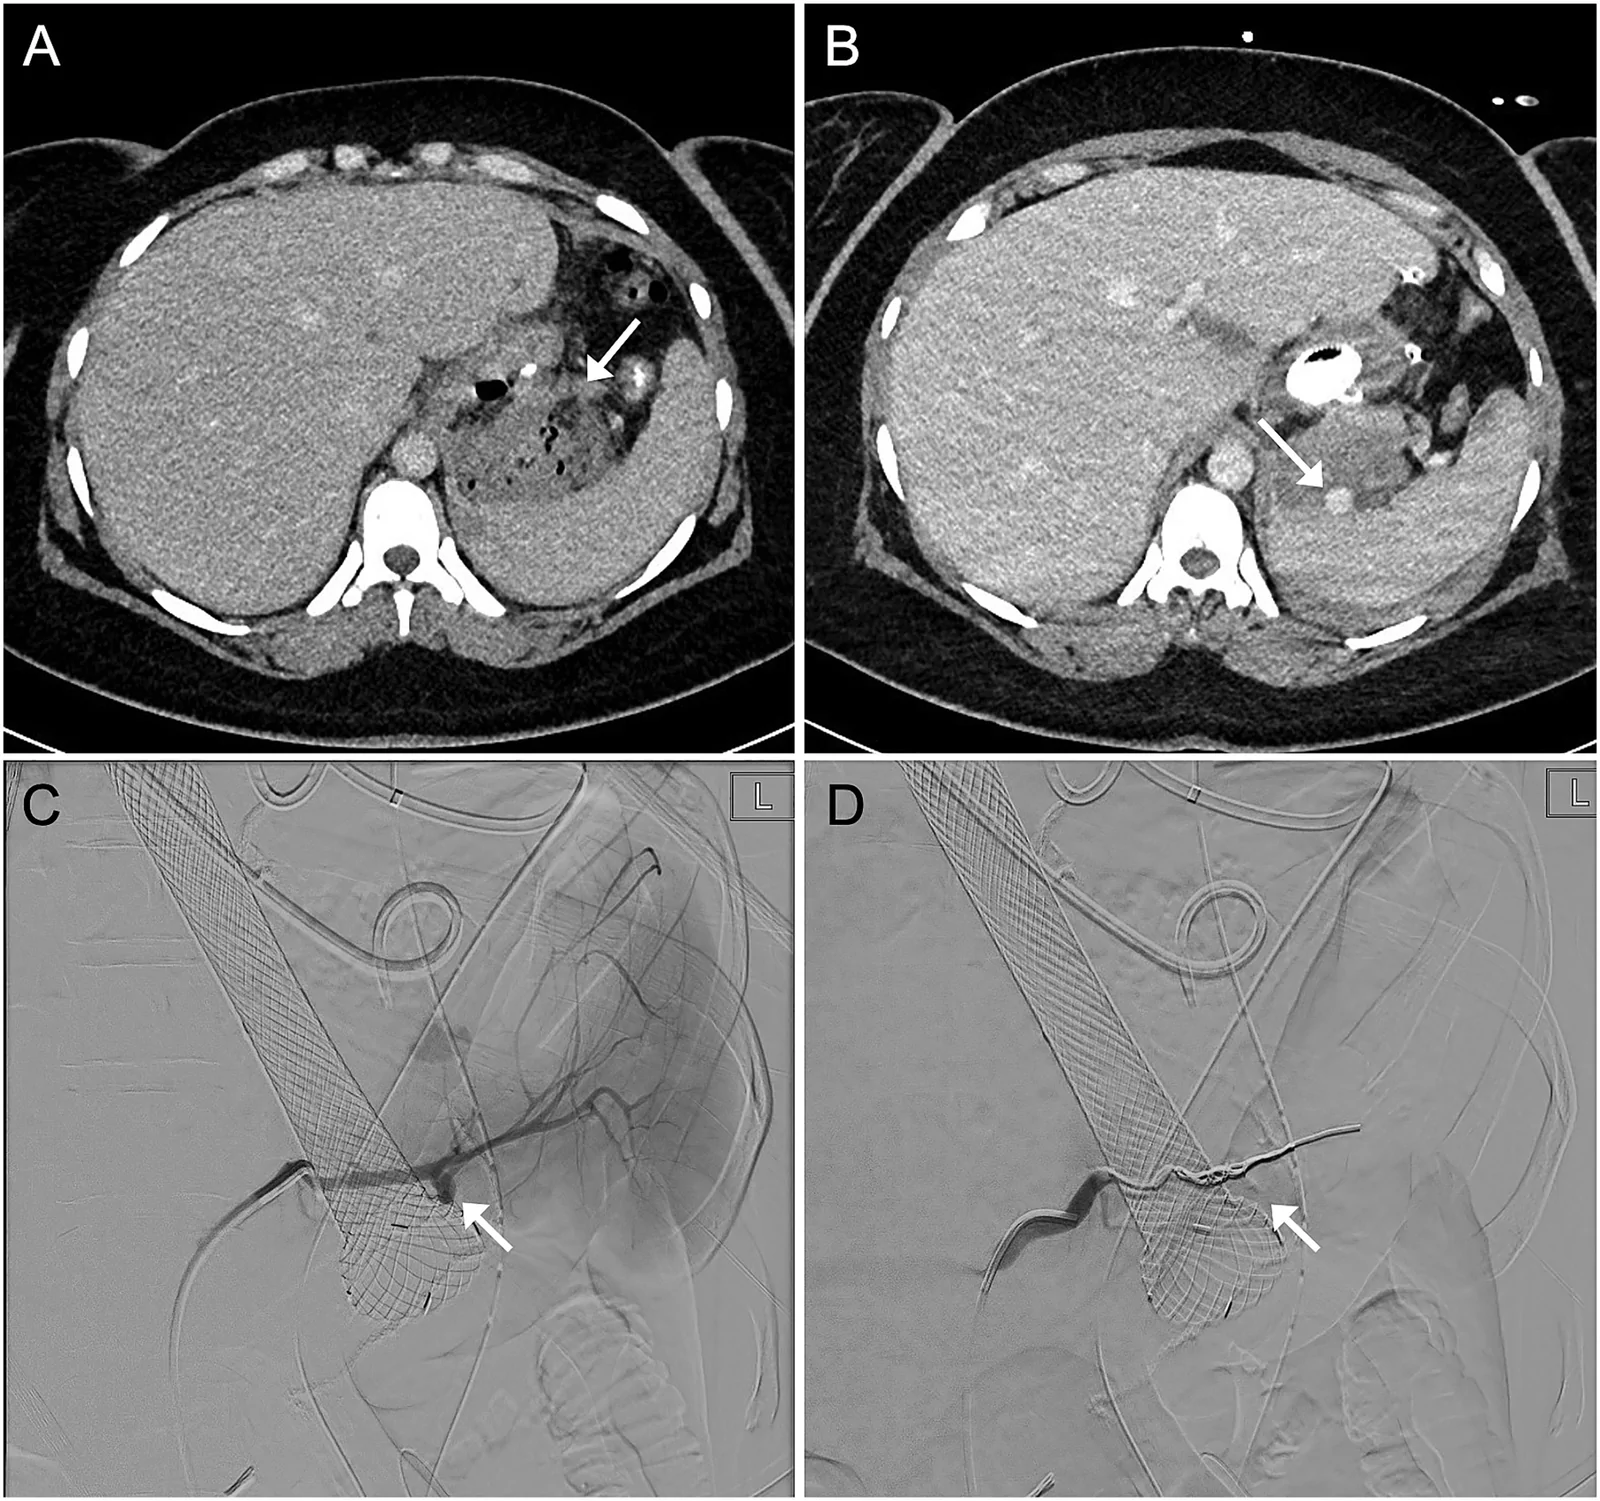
Imaging and endovascular management of splenic artery pseudoaneurysm. (A) CT (POD 13): 7 × 6 × 5 cm gas-containing perigastric collection medial to spleen (arrow). (B) CTA (POD 30): 20 × 18 × 13 mm saccular SAP, distal splenic artery (arrow); no extravasation; splenic perfusion preserved. (C) Pre-embolization arteriography: SAP confirmed (arrow); no active blush. (D) Post-embolization: complete pseudoaneurysm exclusion after proximal coil embolization (arrow).
